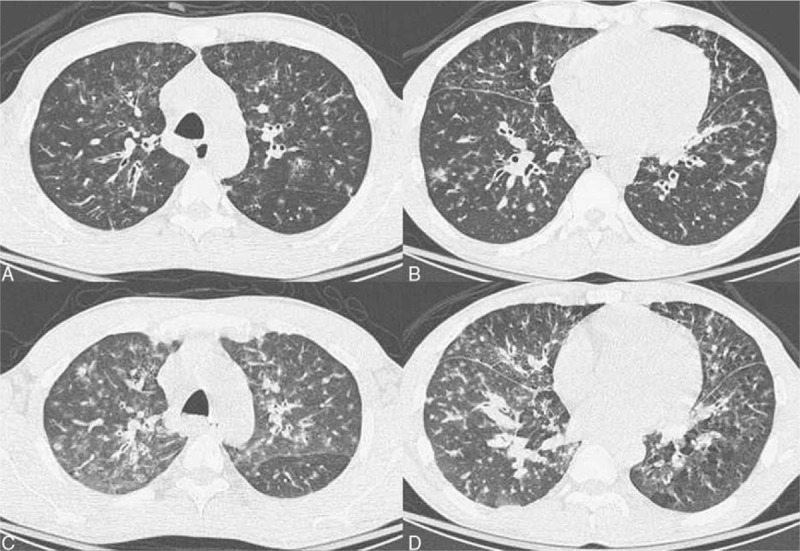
Chest computed tomography during inspiration showing bilateral diffuse centrilobular nodules, tree-in-bud pattern, and bronchial wall thickening (A, B: inspiration). Bilateral multifocal ground-glass opacities and mosaic pattern, indicating air trapping, were observed during expiration (C, D: expiration).
